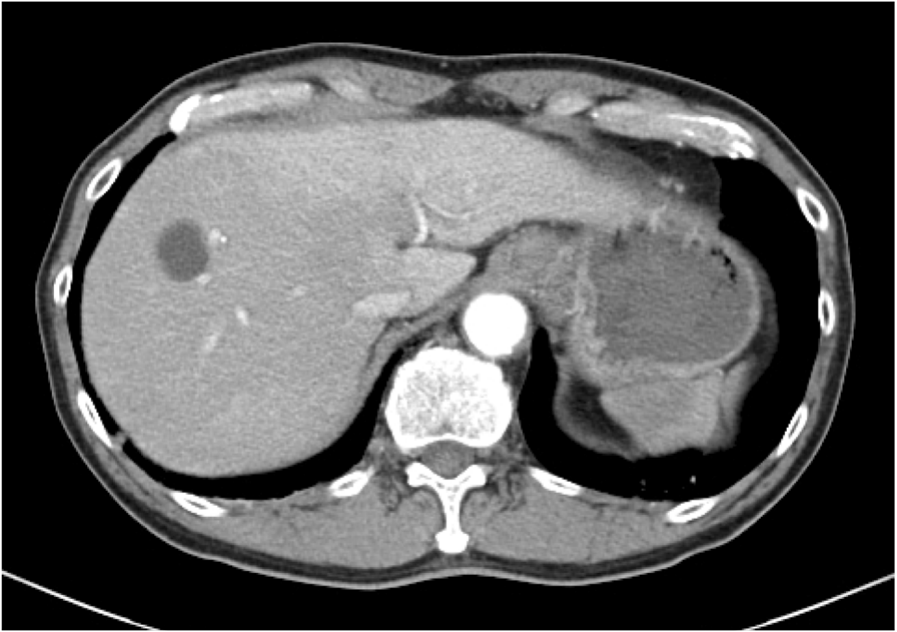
CT 19 days after the operation. CT showed that both hepatic portal gas and pneumatosis intestinalis had disappeared
